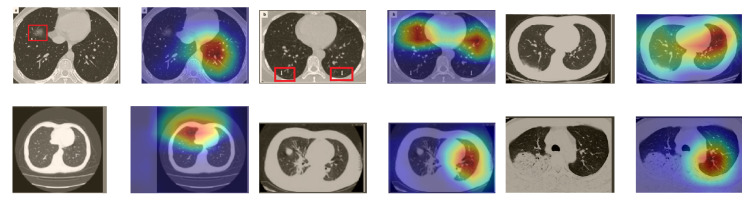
CT scans and their Grad-CAM localization maps showing cases in which the model failed to localize the most relevant COVID-19 regions.
